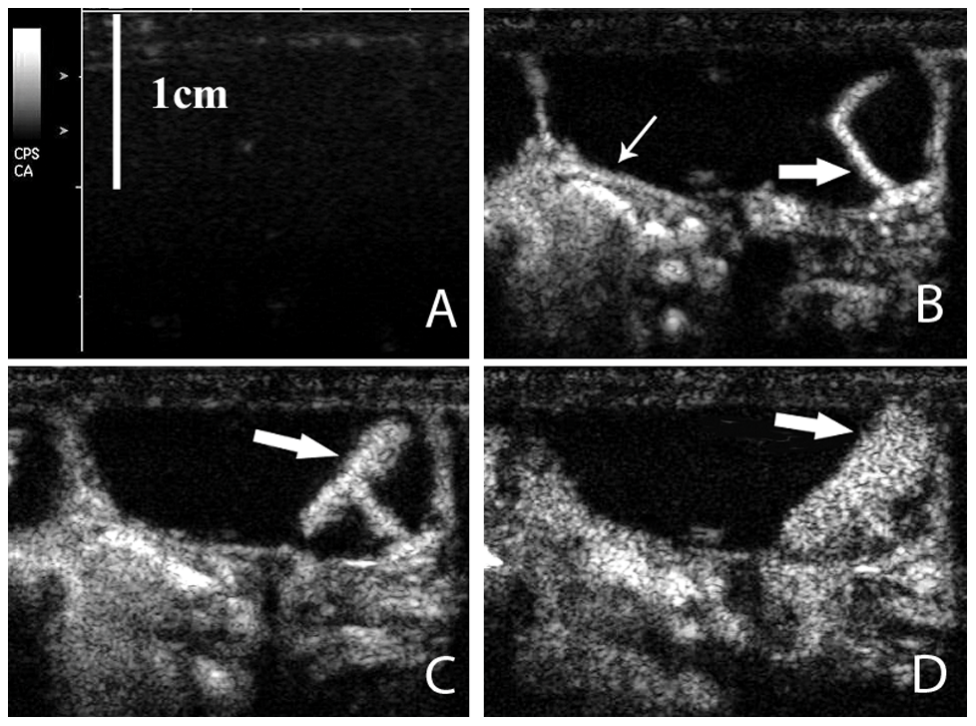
Typical pattern and sequence of placental enhancement after bolus microbubble injection on contrast-enhanced ultrasound (CEUS) with cadence contrast pulse sequencing (CPS) mode (with transmission frequency of 7 MHz, mechanical index <0.2). Before injection, the uterus and placenta could not be visualized (image A). The uterus (small arrow) and central arterial canal (large arrow) were enhanced 4 seconds after microbubble injection (image B). The central potion (→) of placenta was enhanced quickly afterword (image C, 11 seconds after injection). Thereafter, the maternal blood flow percolate back to peripheral portion of maternal side, and the entire placenta (→) was enhanced gradually (image D, 45 seconds after injection).
